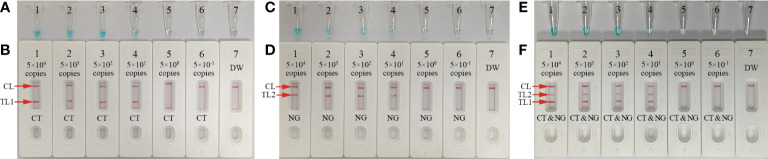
Sensitivity analysis of the mLAMP-AuNPs-LFB assay with serial nucleic acid template dilutions Serial dilutions (5.0×10^4^, 5.0×10^3^, 5.0×10^2^, 5.0×10^1^, 5.0×10^0^, and 5.0×10^-1^ copies) of *C. trachomatis ompA* and *N. gonorrhoeae orf1* plasmids were used as templates, and DW was used as the negative control. Results were simultaneously analyzed by visual reagent malachite green and the AuNPs-LFB. (A, B): A sensitivity analysis of the *C. trachomatis* LAMP assay indicated its LoD was 50 copies per reaction. (C, D): A sensitivity analysis of the *N. gonorrhoeae* LAMP assay indicated its LoD was 50 copies per reaction. (E, F): A sensitivity analysis of the mLAMP assay for *ompA* and *orf1* indicated its LoD was 50 copies of the nucleic acid template per reaction. Key: CL, control line; TL1, test line one; TL2, test line two; CT, *C. trachomatis*; NG, *N. gonorrhoeae*; MG, malachite green.
